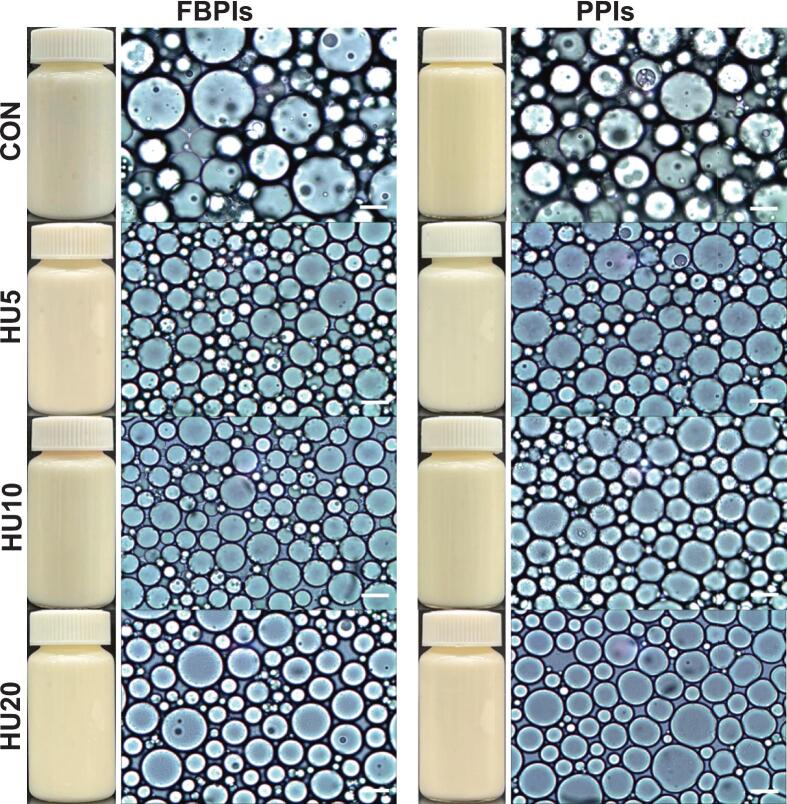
Visual appearance including morphology of HIPPEs stabilized by HU-treated legume protein isolates. HIPPEs, high internal phase Pickering emulsion; FBPIs, faba bean protein isolates; PPIs, pea protein isolates; CON, untreated 4 % protein dispersions; HU, 4 % protein dispersions sequentially heated and ultrasonicated for 5, 10 and 20 min, respectively. Scale bar = 20 μm.
